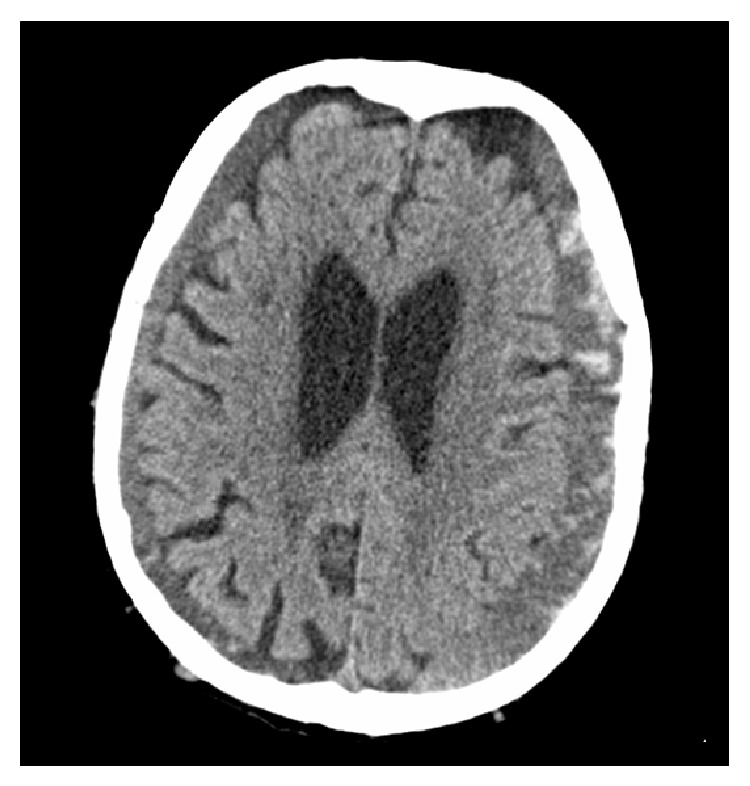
Axial nonenhanced cranial CT scan performed on admission, showing bilateral subdural hematoma with signs of recent bleeding.
